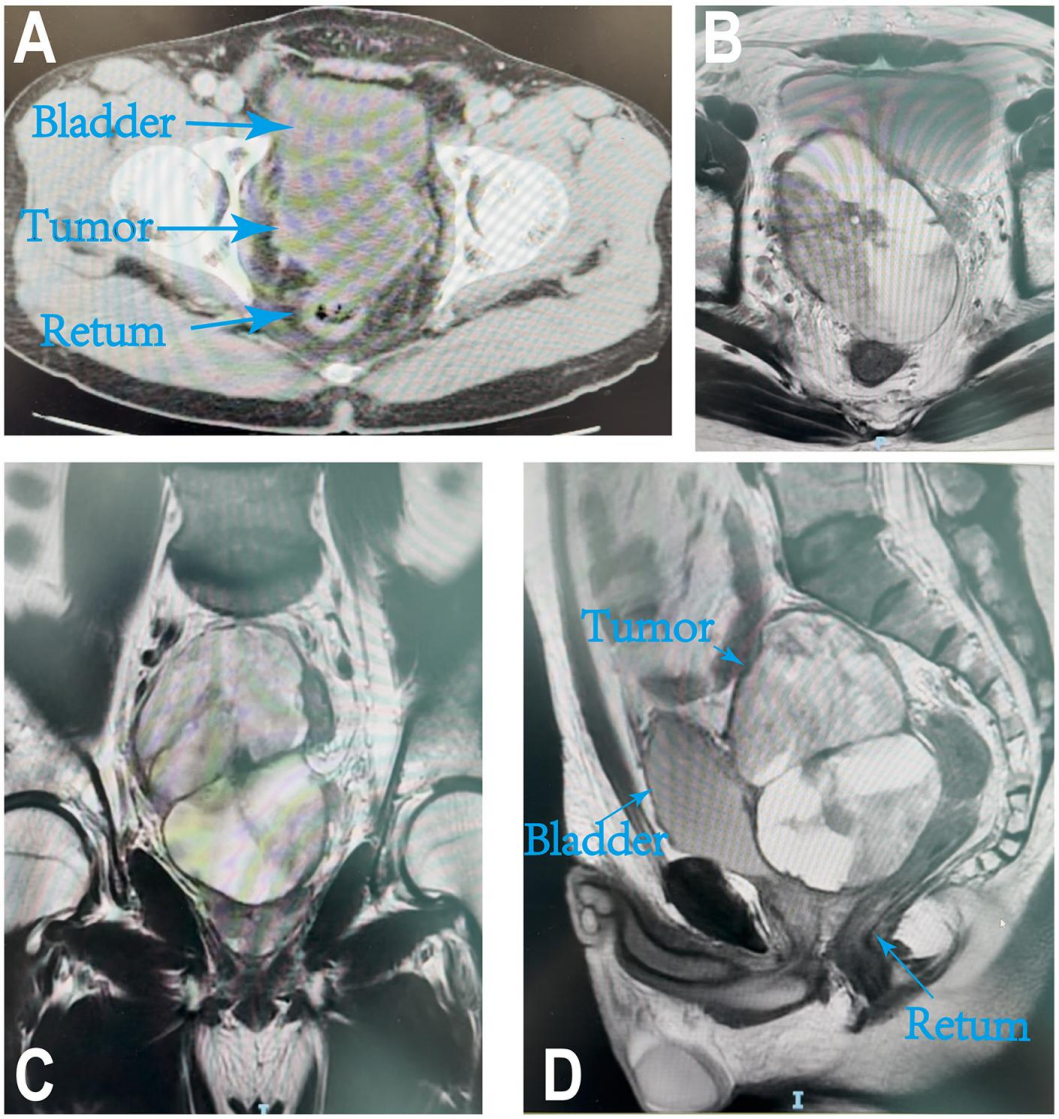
Computed tomography and magnetic resonance images performed at the first hospital. (A) An oval-shaped mass is visible within the right pararectal and retro vesical spaces of the bladder, measuring approximately 9.3 × 6.3 × 7.2 cm in overall dimensions. (B–D) The axial, coronal, and sagittal MRI images revealing a mass located in the pelvic.
